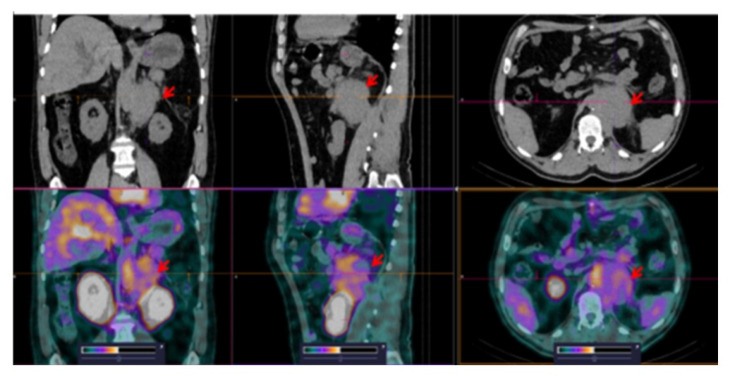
SPECT/CT images of patient 1 at 2 h after injection of [^99m^Tc]Tc-TG. Elevated [^99m^Tc]Tc-TG uptake in the retroperitoneal tumor (SUVmax = 3.92) is visualized (arrows). The upper setting of the scale window (40% of the maximum number) was adjusted to visualize the lesion.
